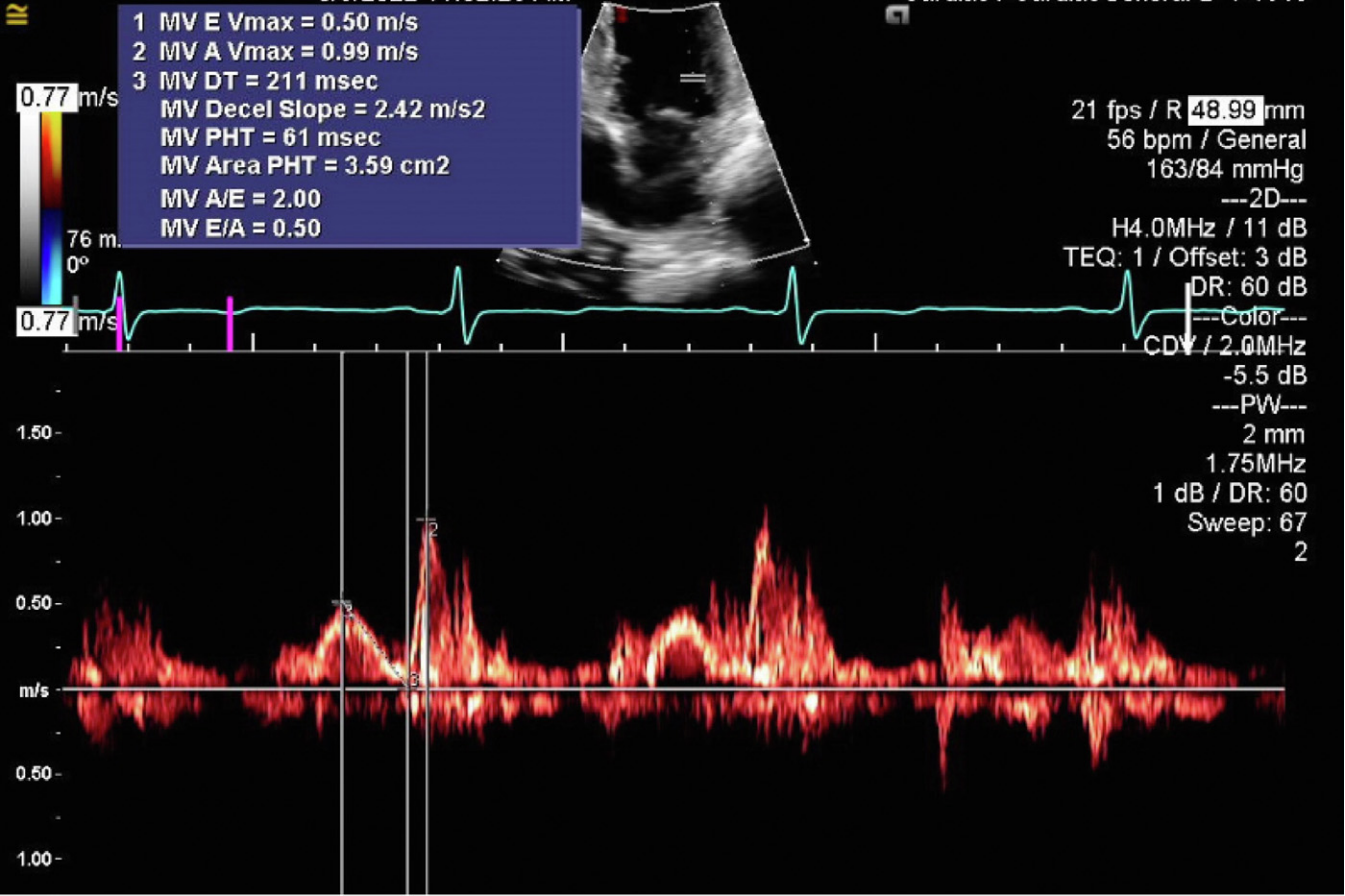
Two-dimensional TTE, apical 2-chamber view, pulsed-wave Doppler at the level of the mitral valve leaflet tip, demonstrating the mitral valve inflow pattern. There is A-wave dominance, suggestive of mild mitral regurgitation.
